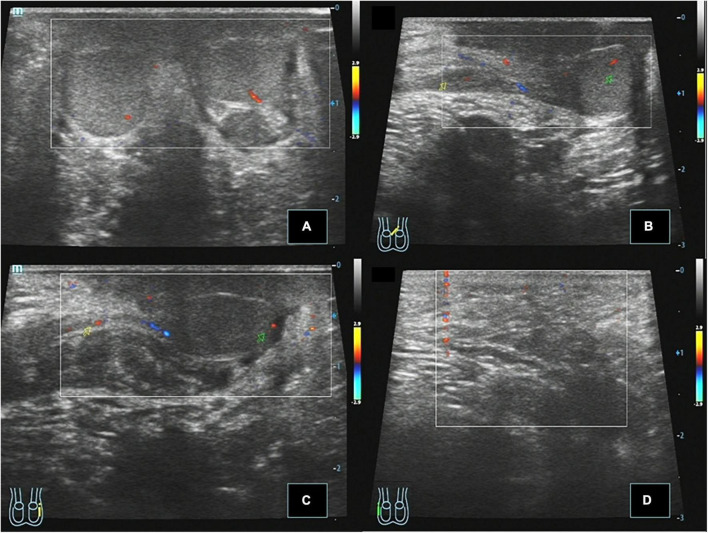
Case 14, 0.8 years, 1 month after Ombredanne operation. Postoperative ultrasound: (A) Longitudinal section of the scrotum showing that both testes were located in the bilateral scrotum, respectively, and CDFI showed that the blood flow signal was normal in the parenchyma. (B) Longitudinal section of the left testis showing that the left spermatic cord continues to the left testis through the left inguinal region. (C) Longitudinal view of the left inguinal region showing that the right spermatic cord echoes across the perineum and continues with the testis in the right scrotum. (D) Longitudinal view of the right inguinal region showing no spermatic cord echo.
